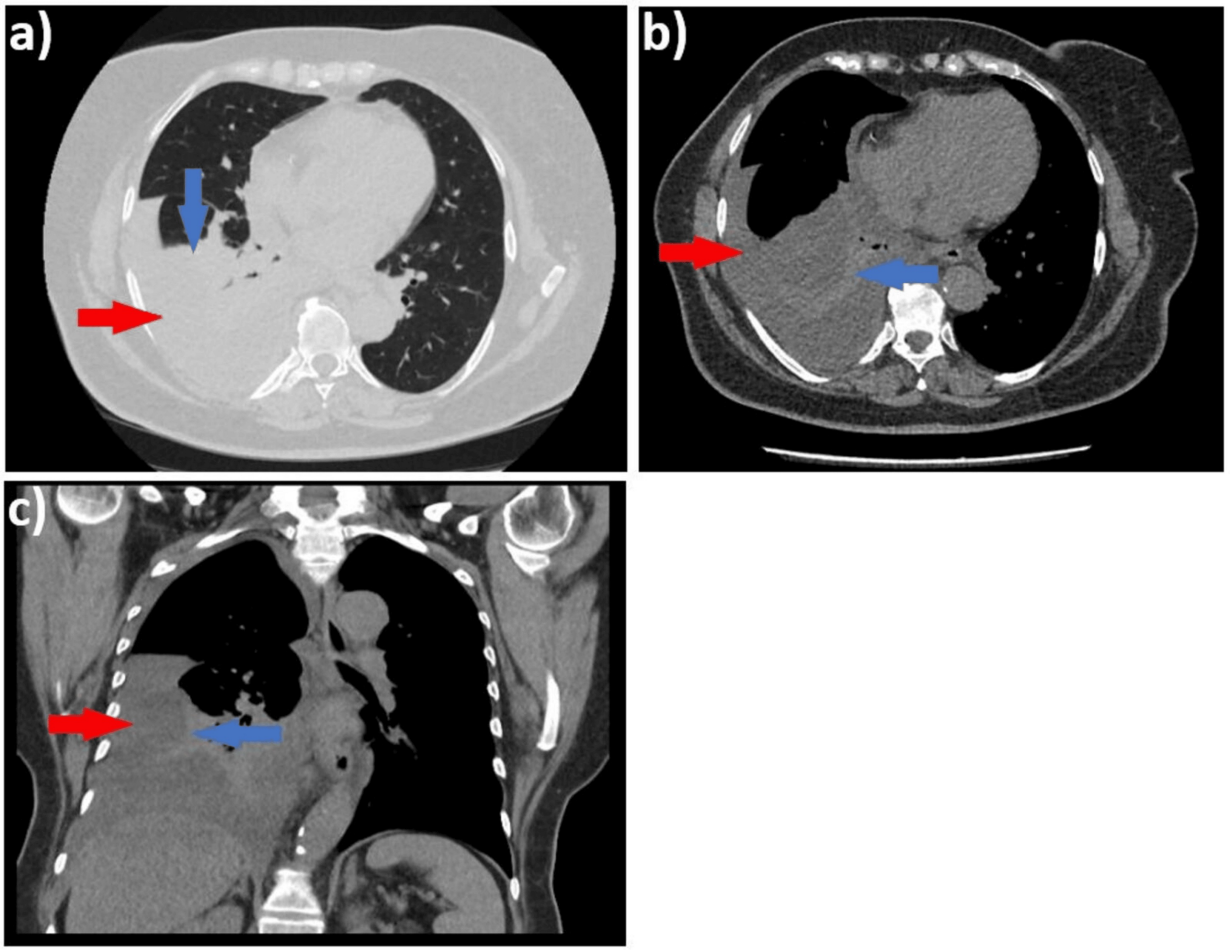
Non-contrast chest CT scan, (a) lung window, axial plane; (b) mediastinal window, axial plane; (c) coronal plane, mediastinal window, showing pleural effusion (red arrows) and collapsed lung (blue arrows)
